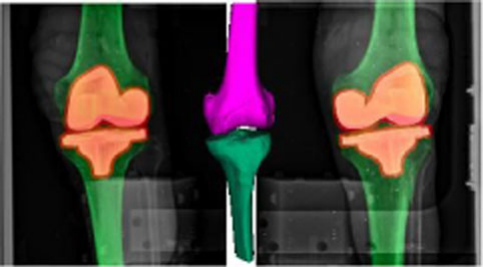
Abbreviations: AutoRSA, AutoRSA software, Orthopedic Research Unit, Aarhus, Denmark; CT, computed tomography; 3D, three‐dimensional; MBRSA, Model‐Based RSA (RSAcore, Leiden, the Netherlands); RSA, radiostereometic analysis.
